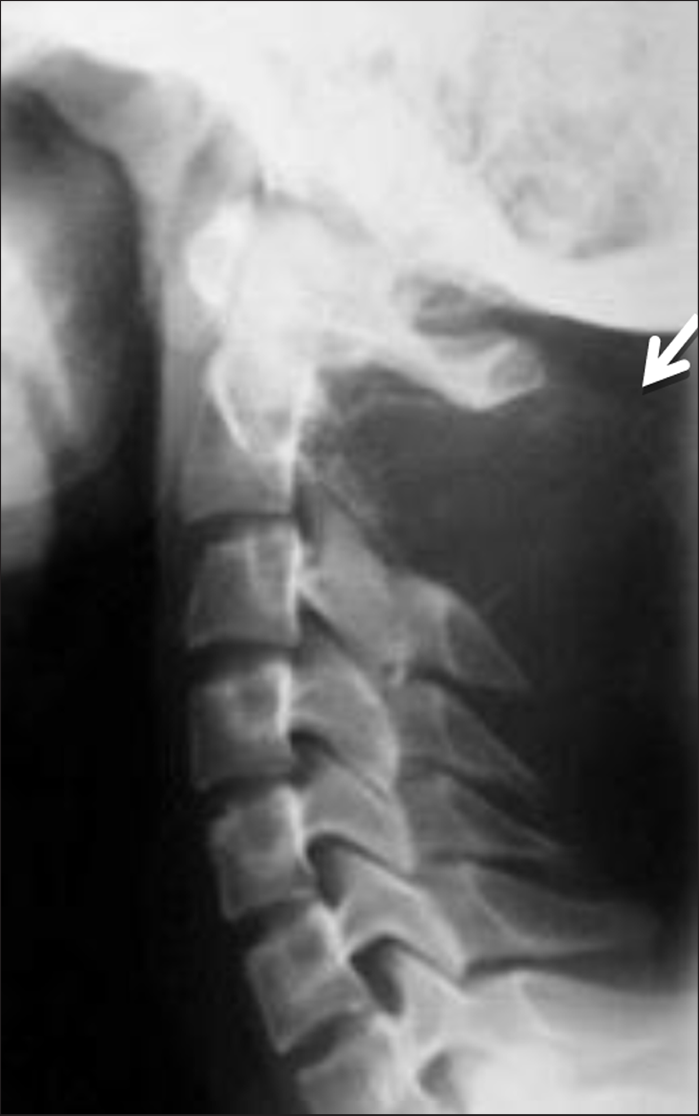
Aneurysmal bone cyst of C2. Lateral radiograph of the cervical spine shows an expansile lesion of the spinous process of C2 (arrow).
